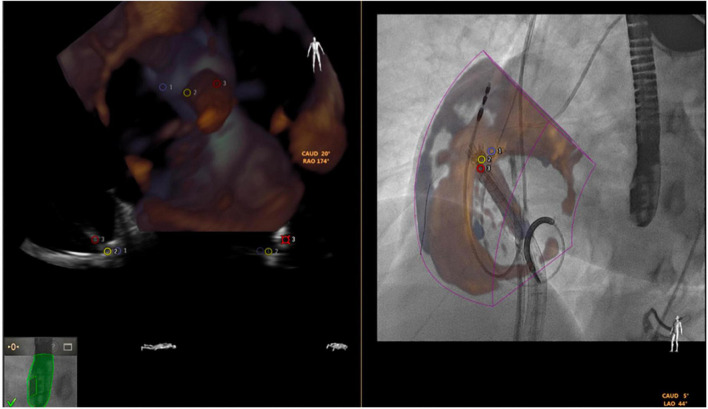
Fusion of 3-dimensional echocardiography and fluoroscopy (using EchoNavigator) in a transcatheter tricuspid Cardioband direct annuloplasty.
